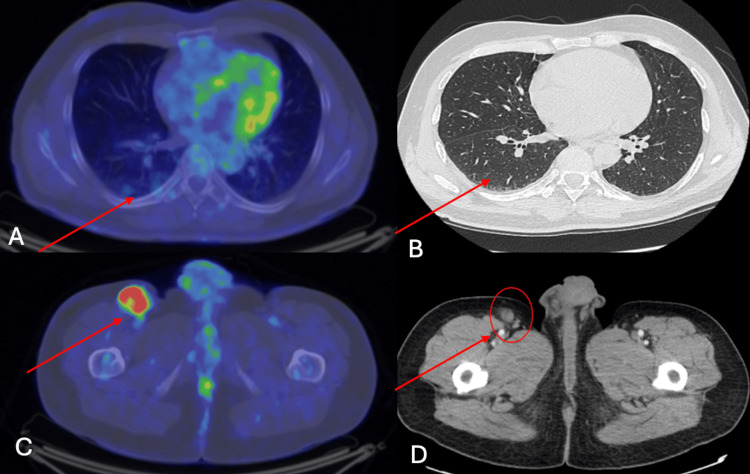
PET-CT at baseline and CT scan reevaluation after ICIs toxicity A: PET-CT baseline - metabolically inactive bilateral lung nodules. B: CT scan - rare lung micronodules - to be monitored. C: PET-CT baseline - confirmed metabolically active right inguinal lymphadenopathy. D: CT scan -  24/17 mm vs 47/33 mm (a decrease of 48.5% of the right inguinal lymphadenopathy).
